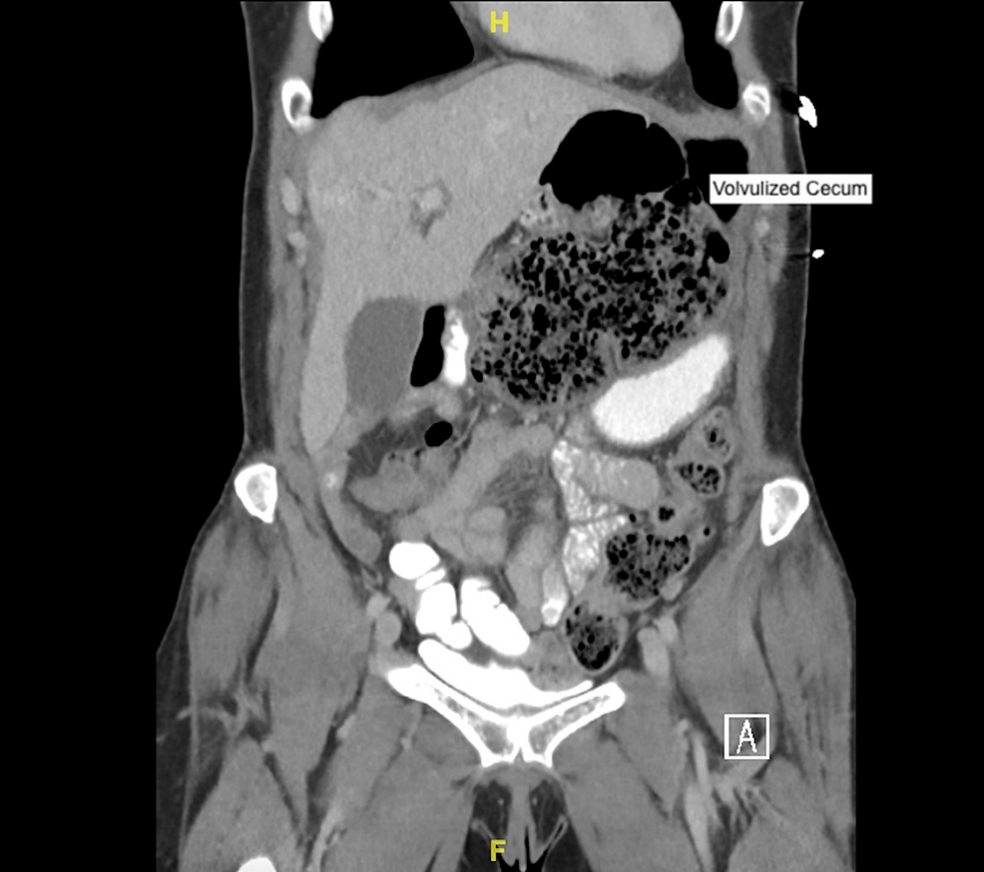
CT of the abdomen and pelvis showing cecal volvulus.
